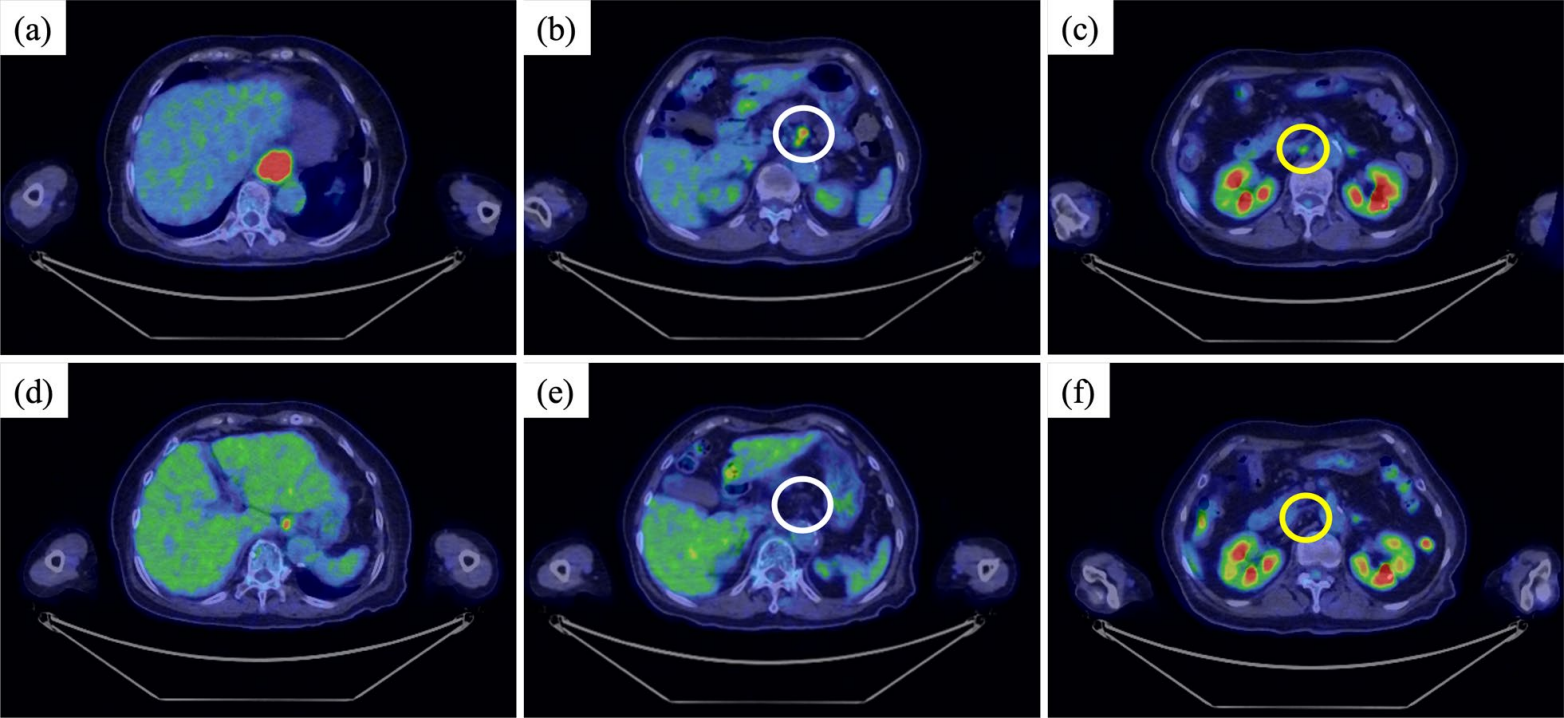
PET–CT. PET–CT reveals the abnormal FDG accumulation in the esophageal wall in the thoracic lower esophagus (a), regional lymph nodes (b, white circle), and the lymph node on the dorsal surface of the inferior vena cava (c, yellow circle). Following preoperative treatment, the abnormal FDG accumulation in the esophageal wall in the thoracic lower esophagus (d), regional lymph nodes (e, white circle) and the lymph node on the dorsal surface of the inferior vena cava (f, yellow circle) disappeared
